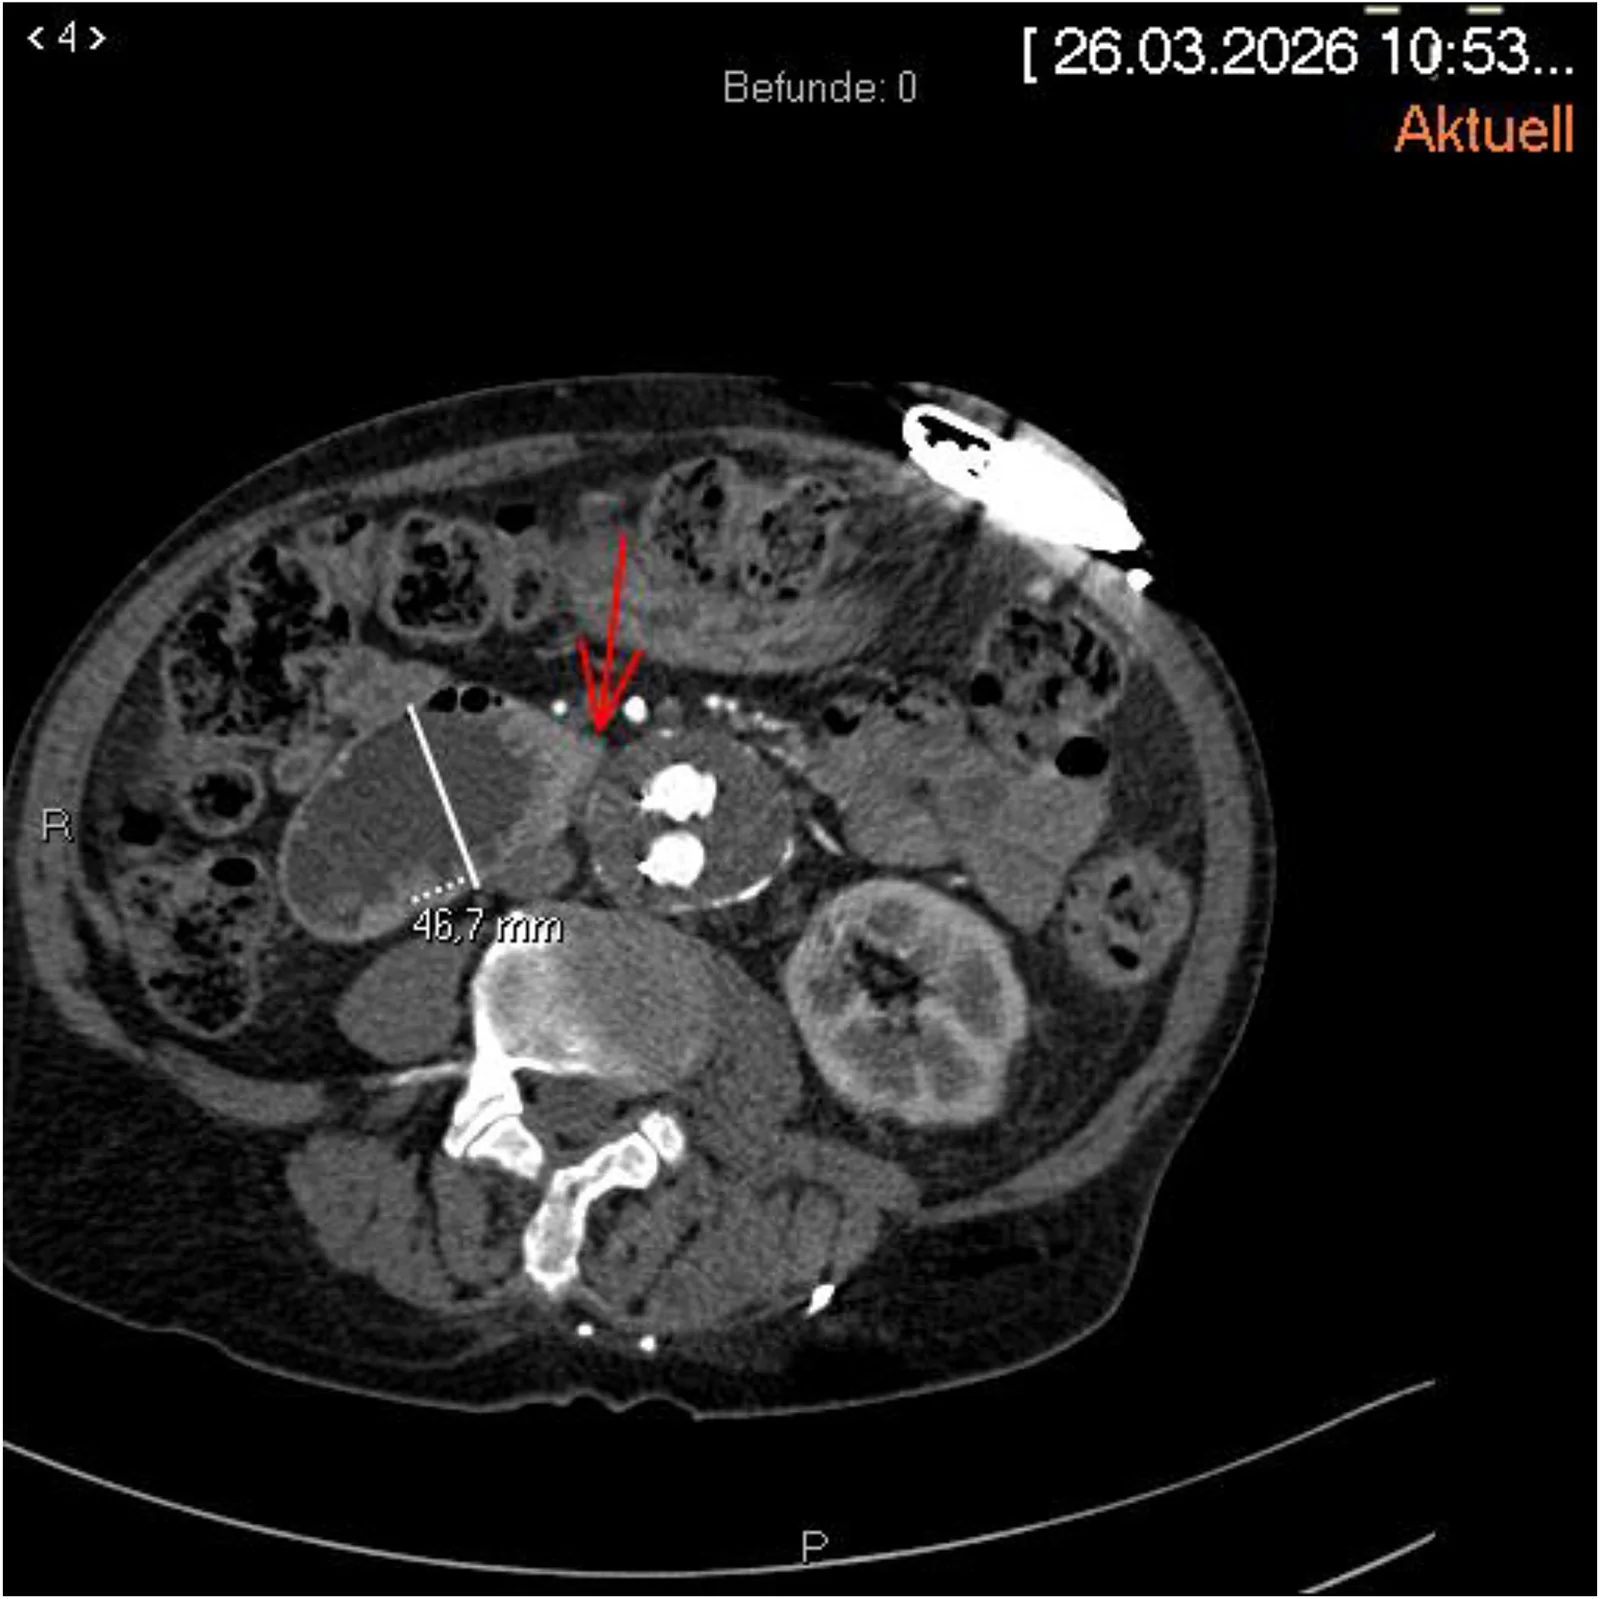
Axial contrast-enhanced CT image from 2026 demonstrating marked upstream duodenal dilatation, measuring up to 46.7 mm, with an abrupt transition at the third portion of the duodenum (red arrow) adjacent to the infrarenal abdominal aortic aneurysm, consistent with aortoduodenal syndrome.
